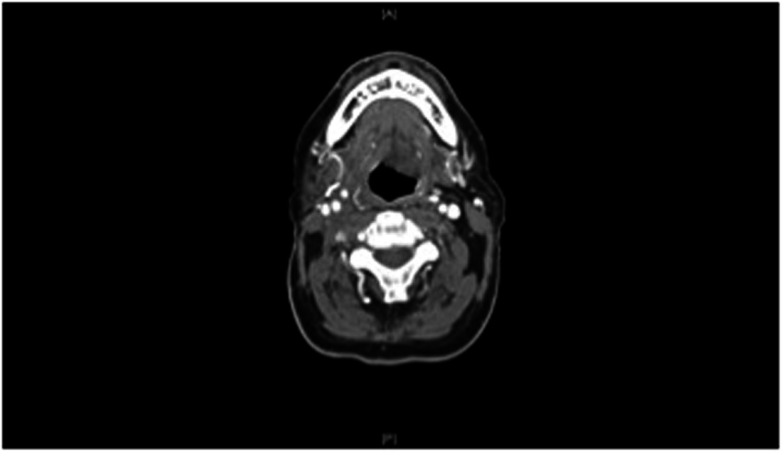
After a 2-week course of stereotactic radiation, CT scan of the neck with contrast showing complete response to therapy.
